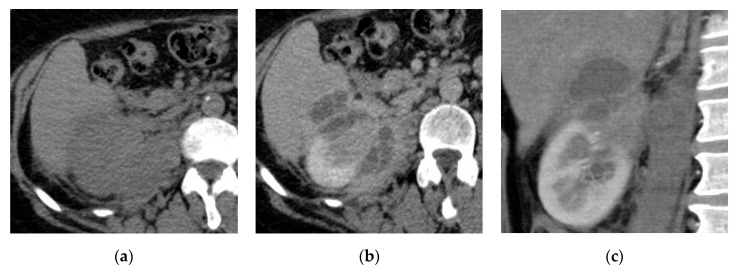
Right renal abscess with hepatic extension. (a) Axial non-contrast CT image shows right upper polar isodense lesion with irregular renal contour. (b) Axial and (c) coronal reformatted post-contrast CT images show multilocular abscess extending to the perirenal space and the liver.
